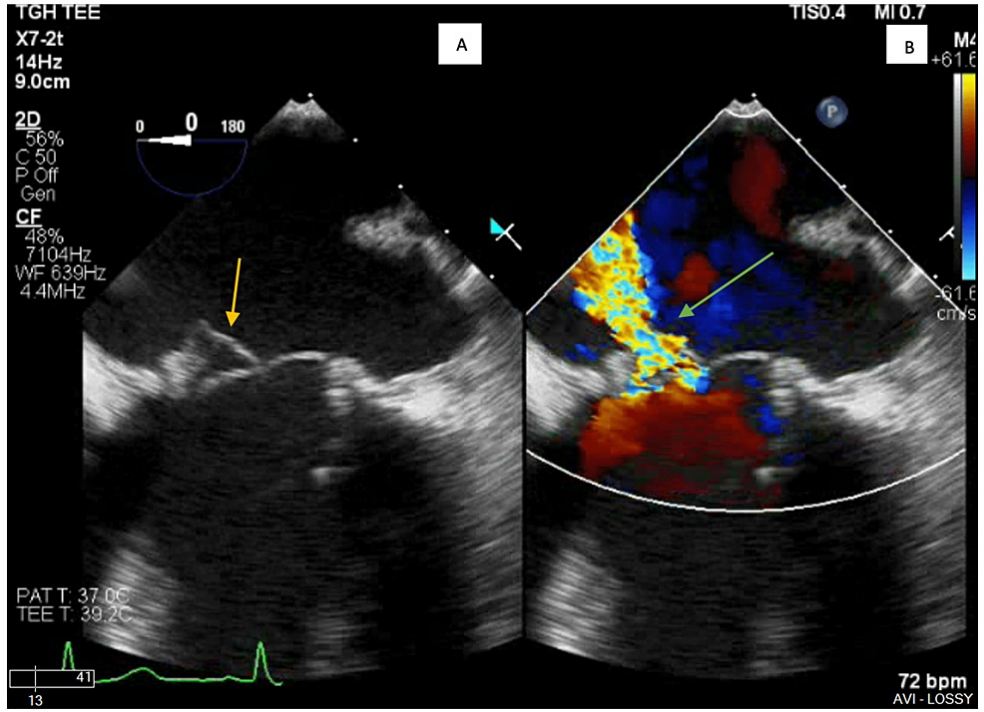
A) TEE of the bioprosthetic MV with flail anterior prosthetic leaflet (yellow arrow). B) Severe eccentric mitral regurgitation (green arrow). TEE: Transesophageal echocardiogram; MV: Mitral valve
